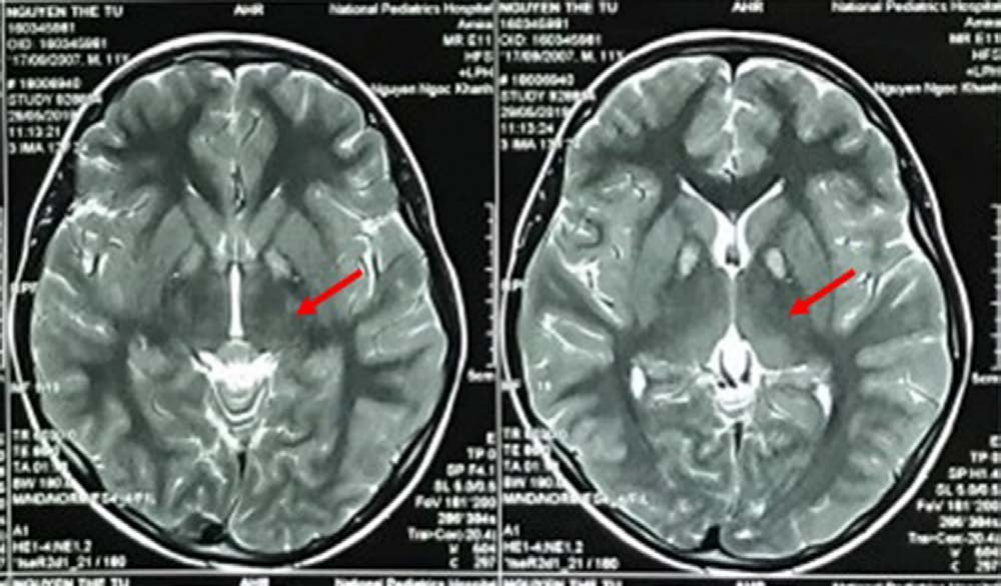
T2 weighted MR imaging in the patient with the “eye of the tiger” sign, which is a characteristic feature of pantothenate kinase-associated neurodegeneration, showed an area of marked hypointensity in the globus pallidus.
